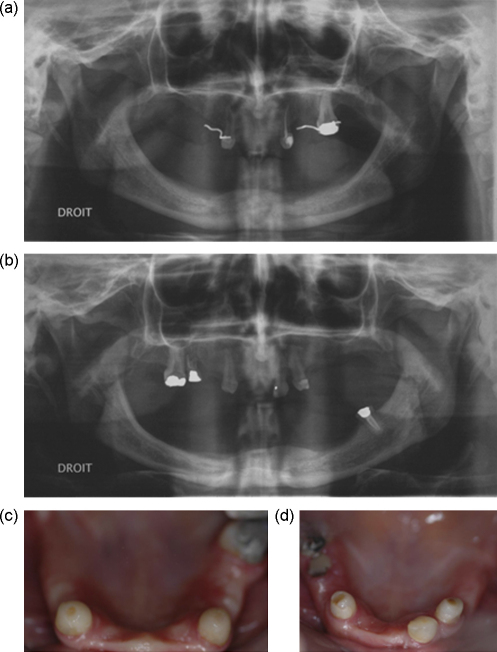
Dental panoramic radiographs (a and b) and maxillary clinical views (c and d) of a pair of monozygotic twins affected by X-linked hypohidrotic ectodermal dysplasia (XLHED) with a mutation of the ED1 gene, coding for ectodysplasin (EDA) morphogenetic factor.
